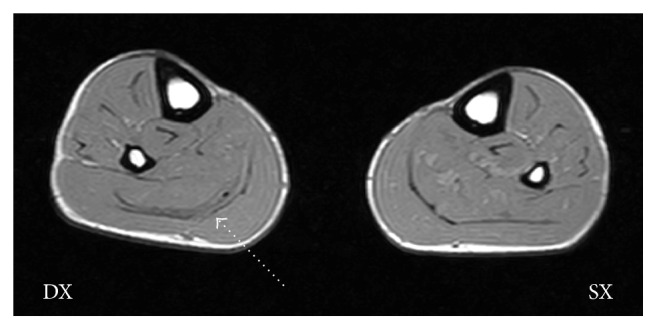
MRI axial section, performed two months after the injury. The* arrow* shows the thickening scar of the right Crural Fascia. Within the scar tissue, intrascar liquid residue, visible as a black dot, is detectable.
